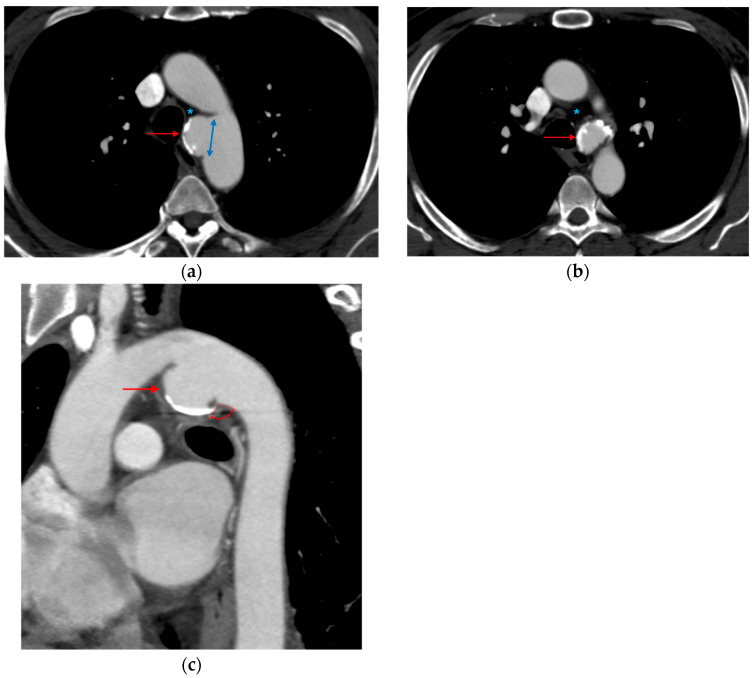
Ductus diverticulum aneurysm. (a,b) Axial CTA images show a well-defined smooth outpouching (red arrows) with a wide base (blue double arrow) and peripheral calcification with preservation of surrounding fat planes (blue asterisk). (c) The sagittal CTA image localizes the outpouching arising from the distal transverse aortic arch at the level of the ligamentum arteriosum (red arrow). The structure shows obtuse angles (red dotted curved line) between the outpouching and the distal aortic wall. There is no evidence of mediastinal/periaortic hematoma or an intimal disruption, which suggests a ductus diverticulum rather than a pseudoaneurysm.
